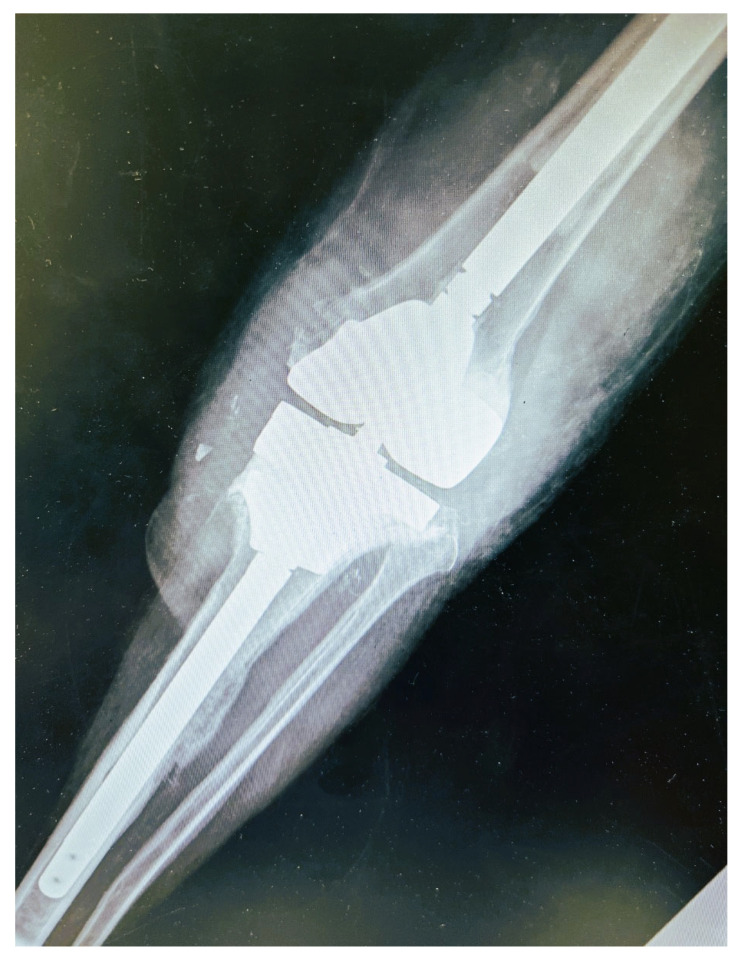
Post-operative anteroposterior X-ray view. A constrained prosthesis with long femoral and tibia stems was placed. A tantalum porous scaffold was placed in the proximal tibia due to bone loss, while augments supported the femoral and tibia components.
